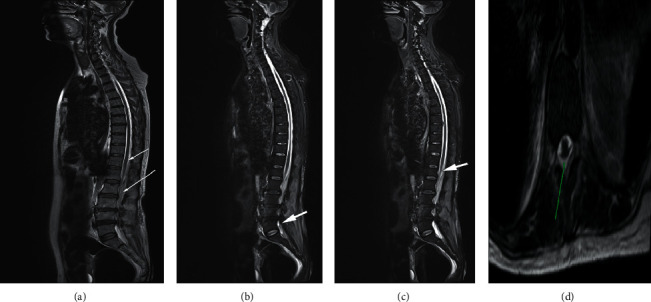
T2-weighted MRI of the spine from the cervical to lumbosacral region depicting a long-segment epidural abscess extending from T11-T12 to L5-S1. Size: 0.8 cm (AP diameter) × 22.5 cm (length) × 1.79 cm (width). No pre- or paravertebral abscesses were visible. (a)-(c): sagittal views. (a): short white arrow- abscess seen commencing at the T12 level and long arrow- abscess extending downwards. (b) and (c): white arrows- abscess visible at L5 segment and the abscess causing cauda equina compression at the L1 level, respectively. (d): axial view; green arrow- abscess at the L4 level.
